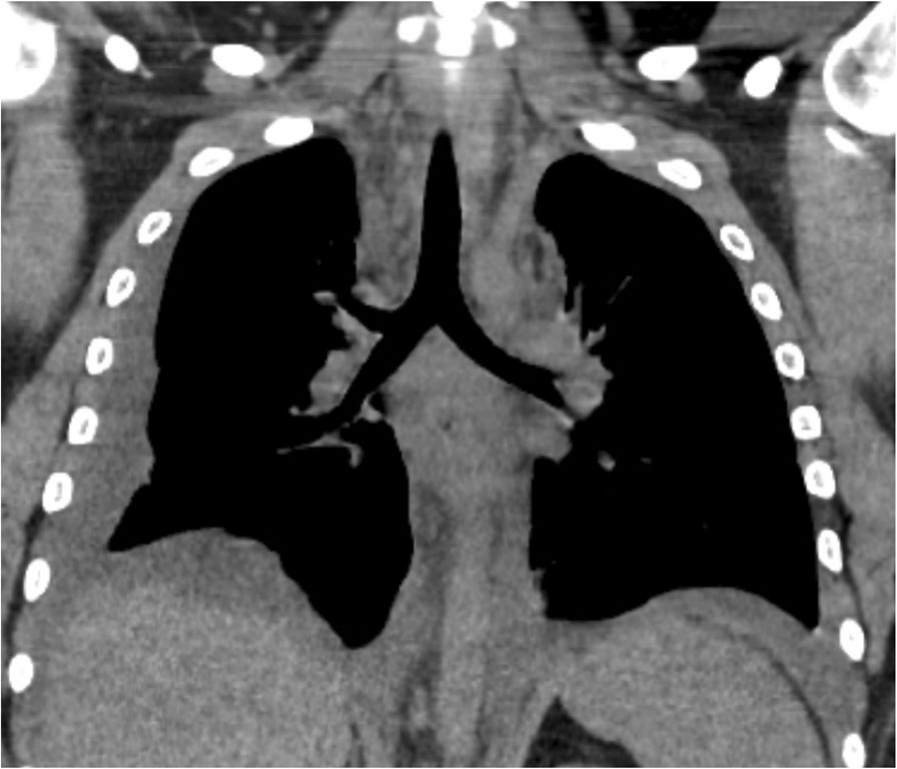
Chest CT scan of the initial presentation of the mediastinitis, with diffuse mediastinal infiltration
